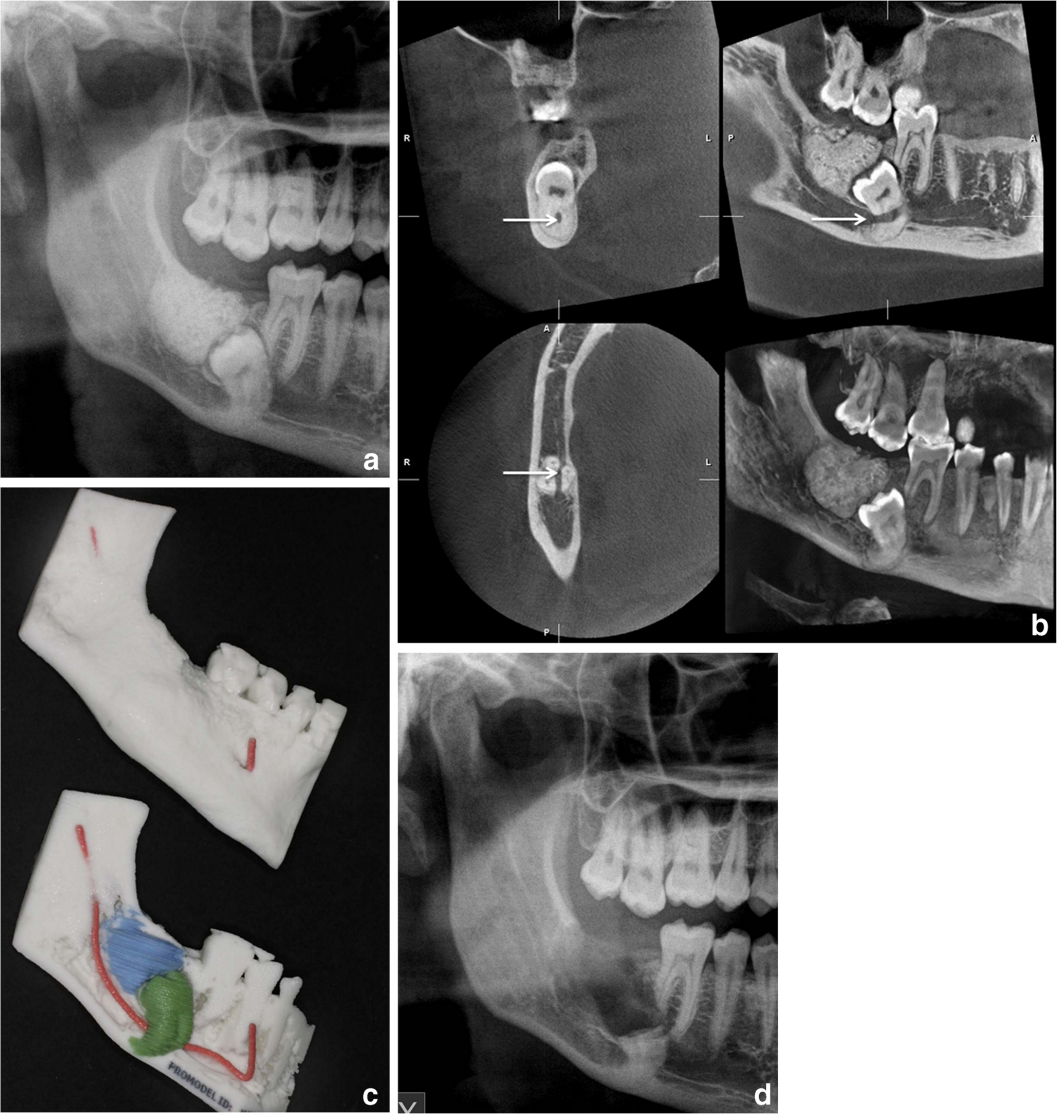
a Cropped PTG shows mesiocaudal dislocation of d. 47 with the root tips projecting into the lower cortex of the mandible. Distocranially to the crown of d. 47 a large complex odontoma is visible. b Based on the CBCT examination the mandibular canal could be located between the buccal and lingual roots (*arrows*); the root tips are in contact apically. c Rapid prototyping models based on the CBCT examination. d Postoperative cropped PTG: the roots of d. 47 were not removed in order to avoid nerve damage of the mandibular nerve bundle
